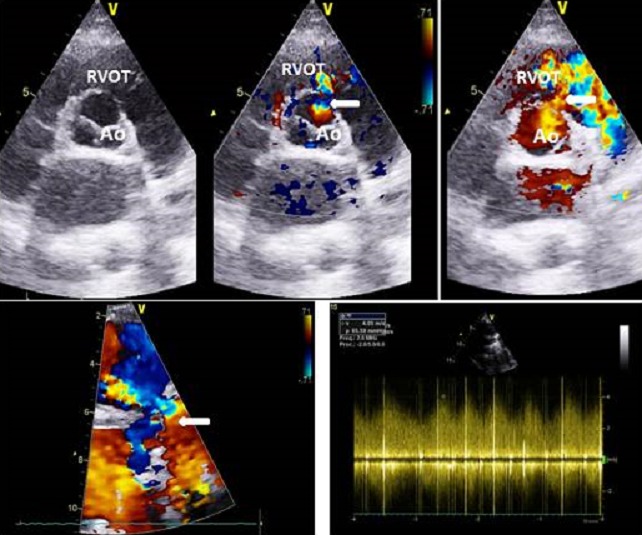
2D-TTE and Doppler interrogation show rupture of the wall of the right coronary sinus of valsalva and flow of blood into the right ventricle
